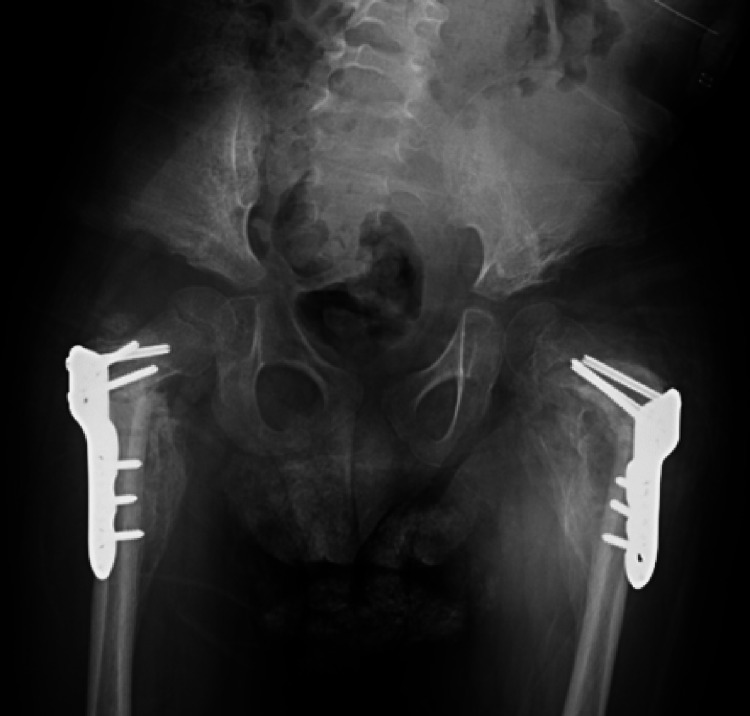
AP radiograph of the pelvis at 10 weeks post revision surgery demonstrating well-located hips, abundant callus formation at the osteotomy sites, and no evidence of implant complication. AP, anterior posterior
